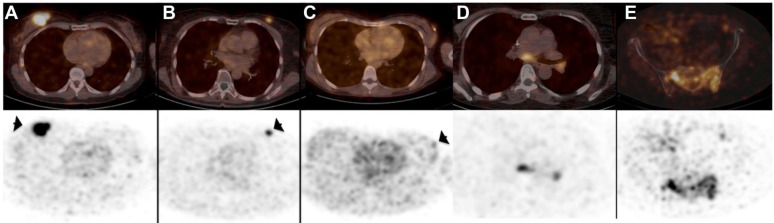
PET/CT scans (top) and PET scans (bottom) after injection of ^68^Ga-HER2-Nanobody showing uptake in primary breast carcinoma lesions (arrows) (A-C) and metastatic lesions in lymph nodes in mediastinum and left hilar region (D) and bone metastasis in pelvis (E). Adapted with permission from 73, copyright 2016.
